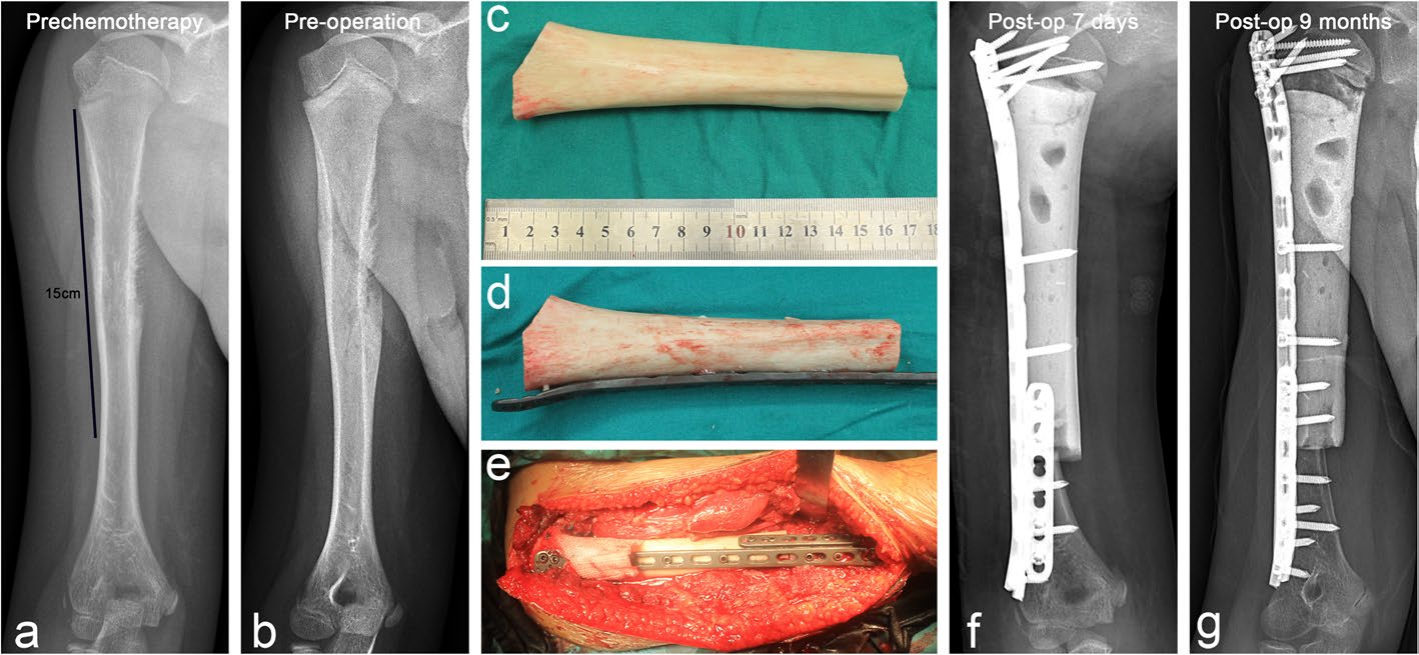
Case 28. A case of stage IIB Ewing’s sarcoma in humeral shaft. a and b Anteroposterior X-rays before and after neoadjuvant chemotherapy. c Allograft for repairing bone defect was filled with bone cement and d fixed with steel plate in advance. e The allograft was combined with the remaining humeral head and distal humerus, and the distal host–donor junction was reinforced with a short plate. f and g Anteroposterial X-rays were performed at 7 days and 9 months postoperatively, respectively
